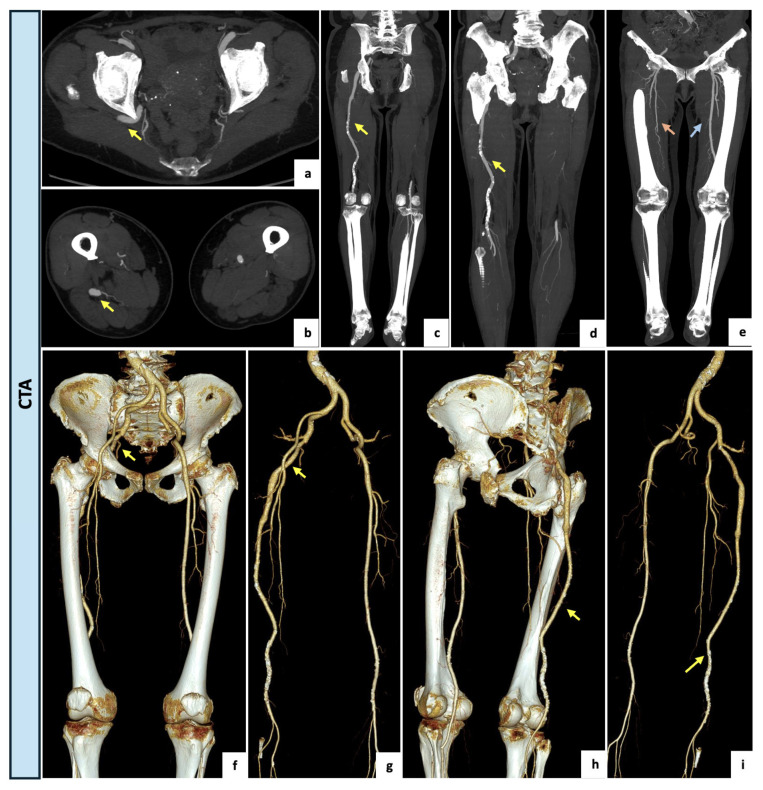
(a–d) Axial and coronal maximal intensity projection views of the CTA demonstrating a right complete and ectatic PSA of 13 mm (yellow arrow) originating from the internal iliac artery and accompanied by an incomplete ipsilateral SFA interrupted at Hunter’s canal (type 2a). (e) Coronal view of lower limbs with a right incomplete ipsilateral SFA (orange arrow) and a regular contralateral femoral axis (blue arrow) (f–i) Three-dimensional reconstructions showing the ectatic PSA (yellow arrow) running through the greater sciatic notch, following the proximal course of the sciatic nerve and continuing as popliteal–tibial axis.
